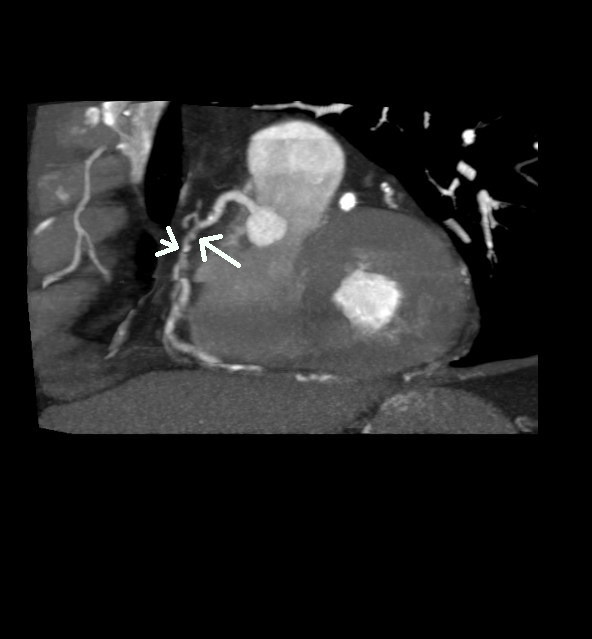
Stenosis of the right coronary artery (arrow). Contrast-enhanced slice CT shows the second segment of the right coronary artery in a multiplanar reconstruction. At the site of lumen reduction, a coronary atherosclerotic plaque with positive remodelling can be seen (large arrow). A small calcification is present toward the distal border of the plaque (small arrow).
